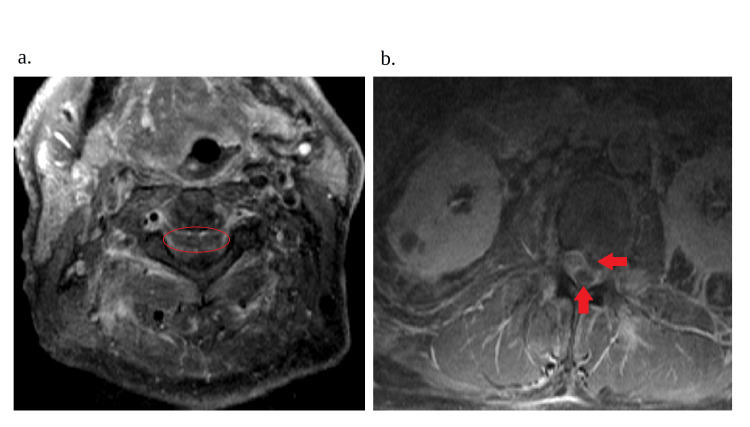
MRI of the spine transverse view 2a. Cervical spine and the red circle indicating a collection in the cervical region of the patient’s spinal cord.2b. Lumbar spine and red arrows showing a collection in the lumbar spine that wraps around the spinal cord.
